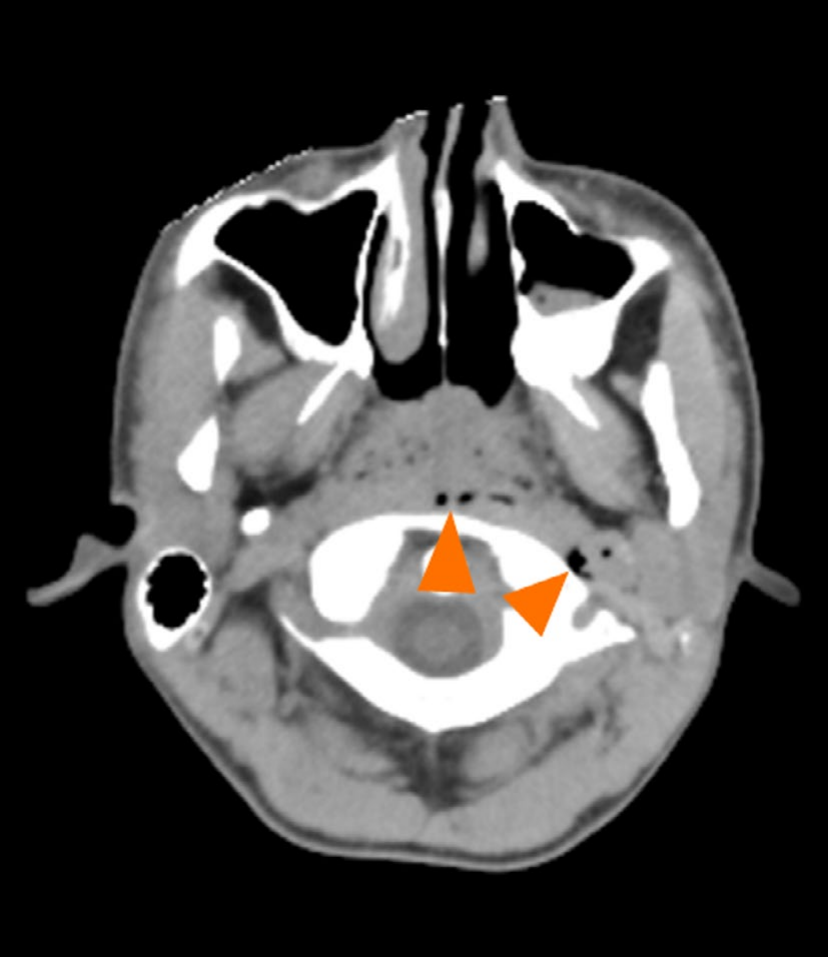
Head computed tomography shows emphysema in the nasopharynx
